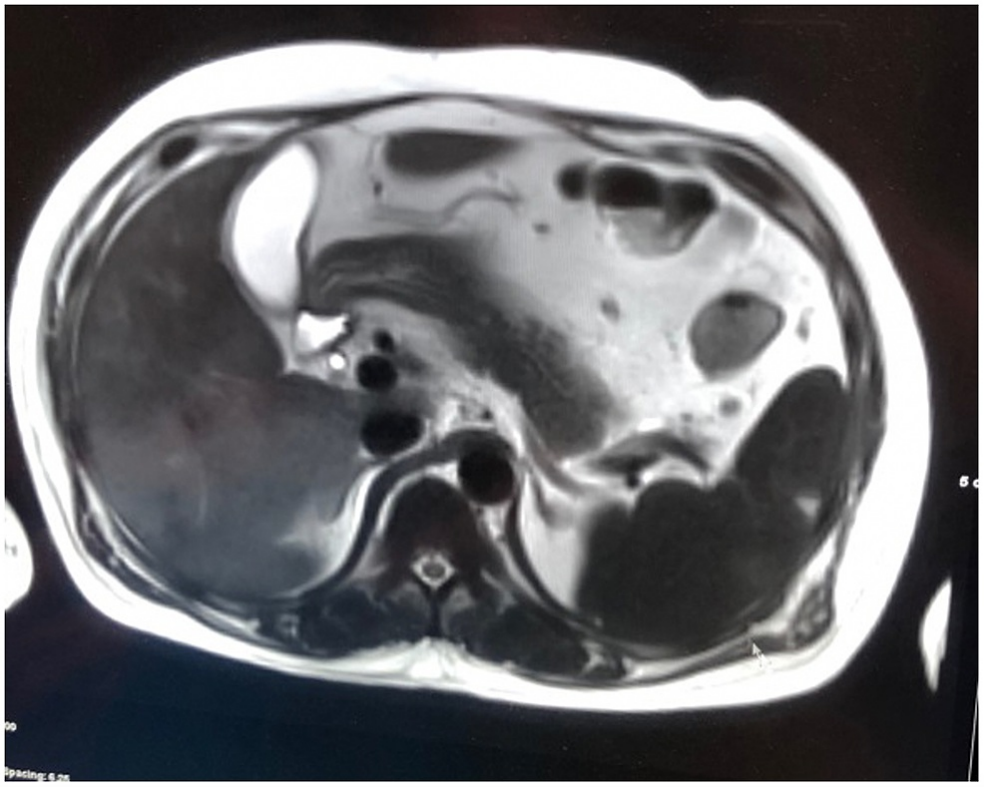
MRI of the abdomen reveals peripheral wedge-shaped defects with decreased signal intensity in the spleen, confirming the diagnosis of splenic infarction.
